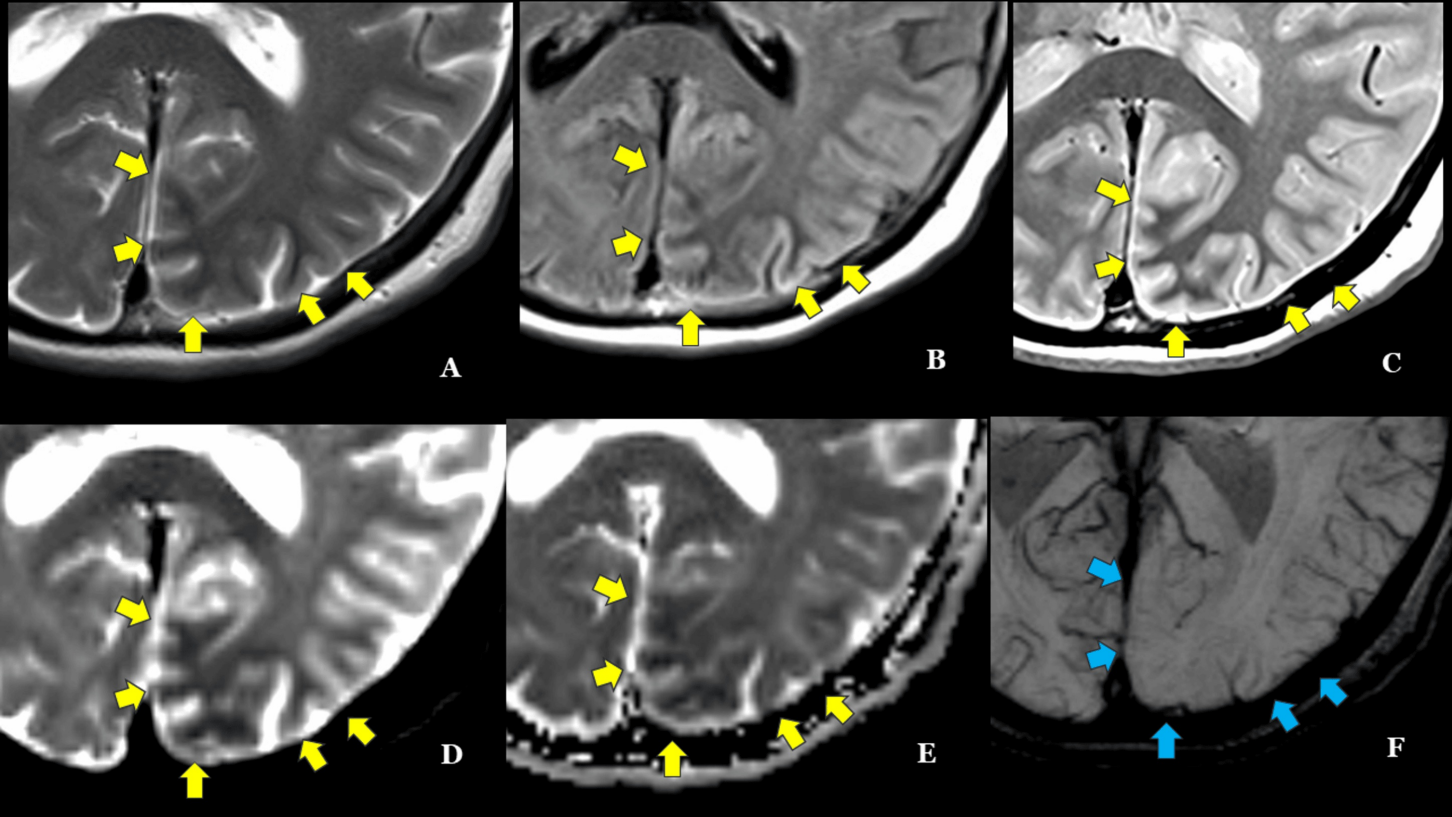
Magnified image of the lesion observed on MRI in Figure 1 (A) T2-weighted imaging, (B) FLAIR, (C) proton-density weighted imaging, (D) b=0, and (E) ADC map; hypointensity is observed in the subcortical white matter (yellow arrows). (F) SWI showing decreased venous visibility (blue arrow). FLAIR: fluid-attenuated inversion recovery; ADC: apparent diffusion coefficient; SWI: susceptibility-weighted imaging
